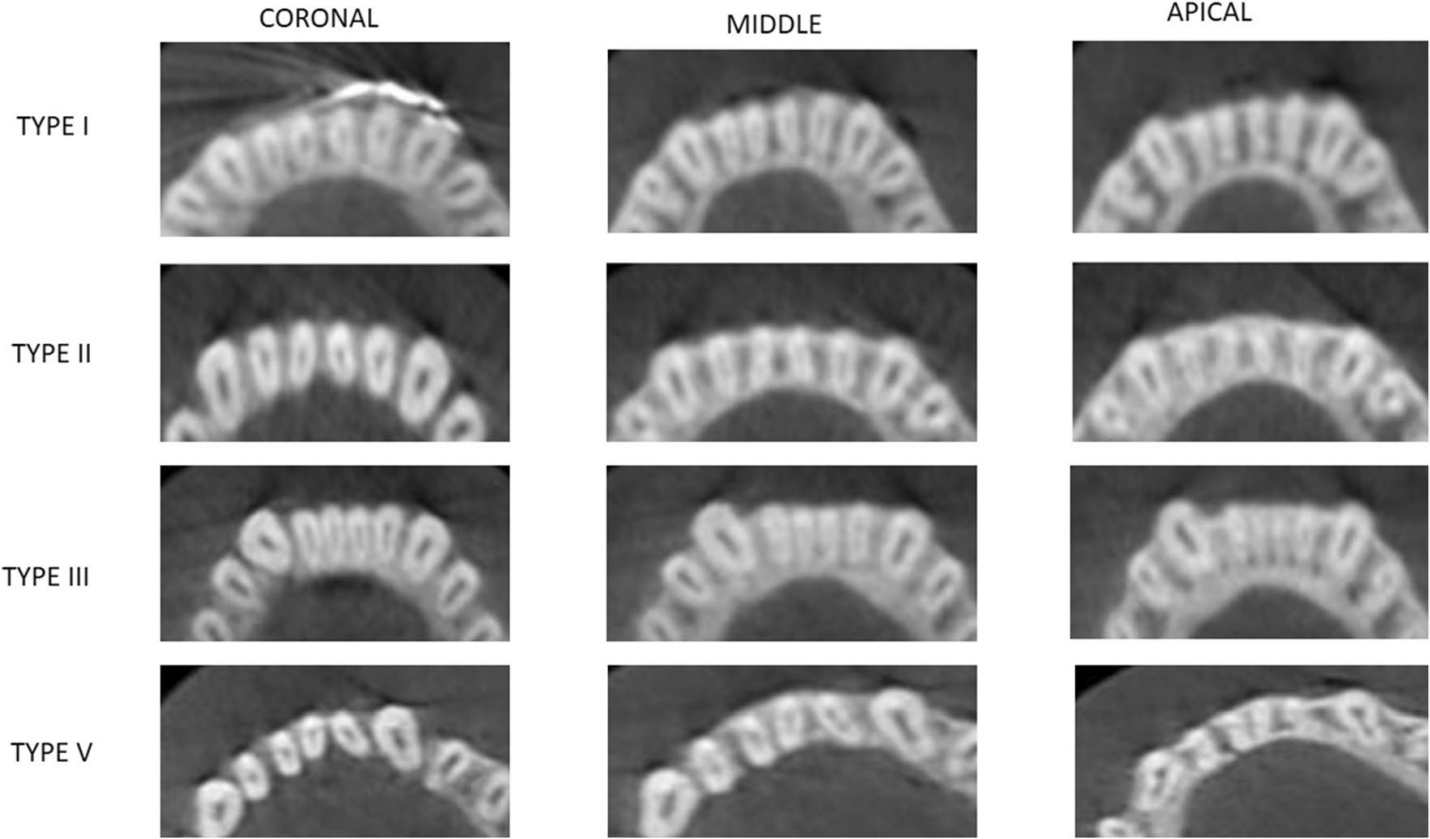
CBCT scans showing root canal morphology according to Vertucci’s classification in Mandibular incisors.
